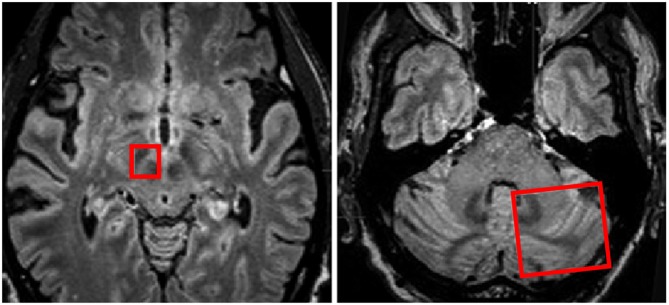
Regions of interest (red boxes) shown on MRI in axial planes to include the right subthalamic nucleus (STN) and Red Nucleus and the contralateral cerebellar hemisphere for investigation of white matter tracts traversing between the two regions of interest. Adapted from Sweet et al. (2014a).
